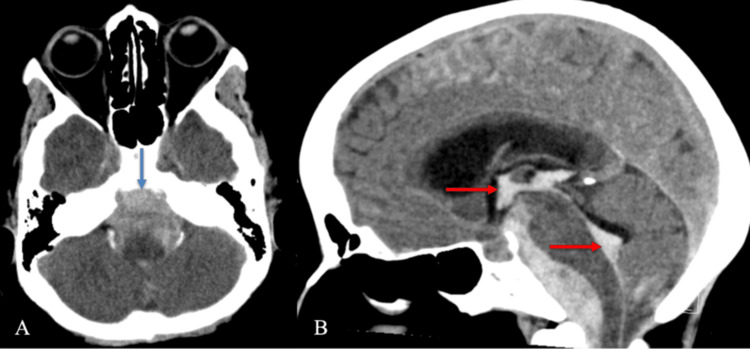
Non-contrast CT head demonstrating diffuse SAH with intraventricular extension (A) Axial non-contrast CT head demonstrates diffuse SAH centered in the prepontine cistern (blue arrow), extending into the basal cisterns and perimesencephalic spaces. (B) ﻿﻿﻿﻿Sagittal non-contrast CT head from the same exam shows intraventricular extension of hemorrhage into the third and fourth ventricles (red arrows), consistent with Modified Fisher grade 4 SAH. Mild ventricular enlargement is present, suggesting early hydrocephalus CT: computed tomography; SAH: subarachnoid hemorrhage
